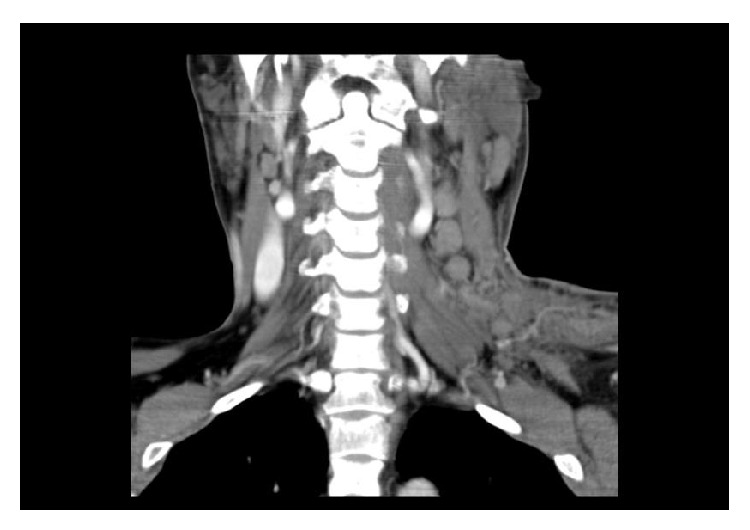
CT coronal reconstruction revealing the presence of diffuse left cervical lymphadenopathy.
